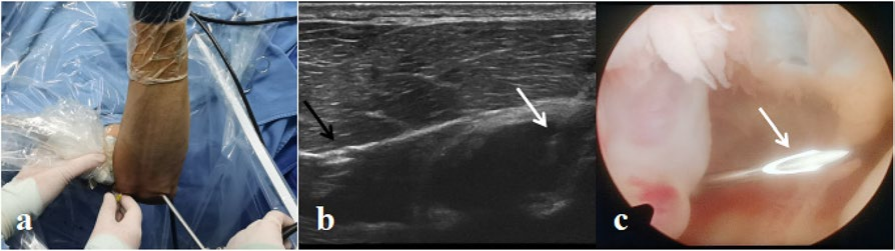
A 41-year-old male in the intraoperative ultrasound group. a Top view image of the right elbow joint with the patient in the supine position. A linear probe is held to view the radial nerve. A pilot needle is slowly inserted inside the anterolateral elbow joint. b The real-time ultrasound image of the radial nerve (black arrow) and pilot needle (white arrow). The needle is inserted into the anterolateral elbow joint. c Corresponding arthroscopic image obtained from the direct lateral viewing portal, showing insertion of the pilot needle (white arrow) into the anterolateral elbow joint
